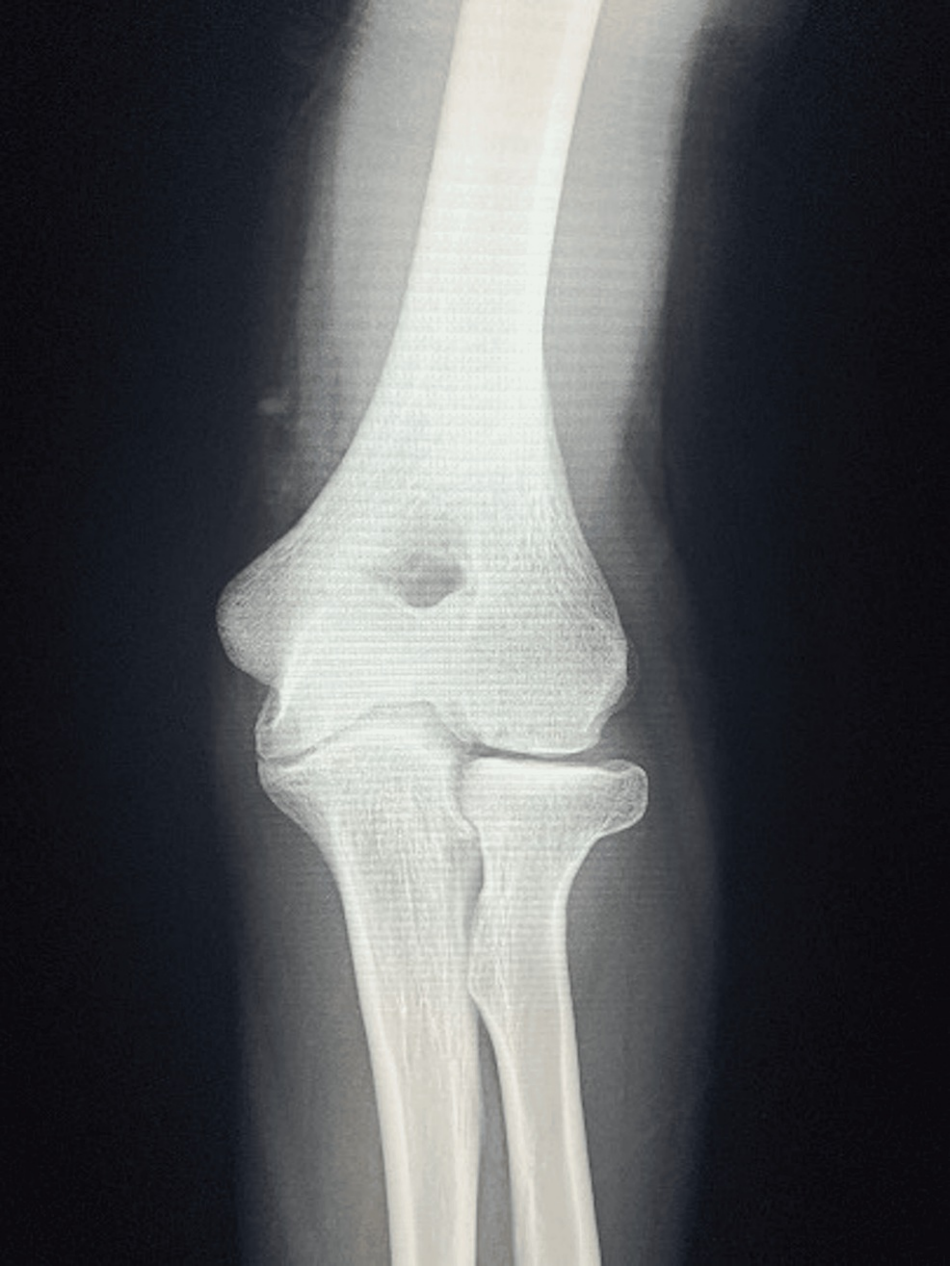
X-ray of the elbow joint 10 years after initial injury. Fishtail deformity due to growth impediment of the lateral part of the trochlea and compensatory hypertrophy of the radial head is evident.
